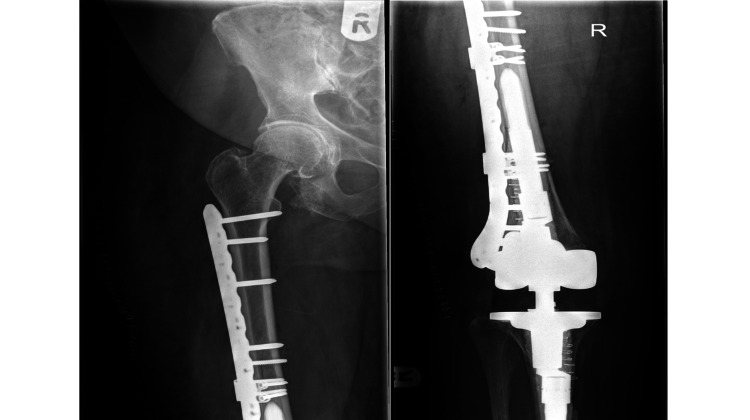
Radiographs for a patient managed with plate fixation (which subsequently failed due to non-union)
